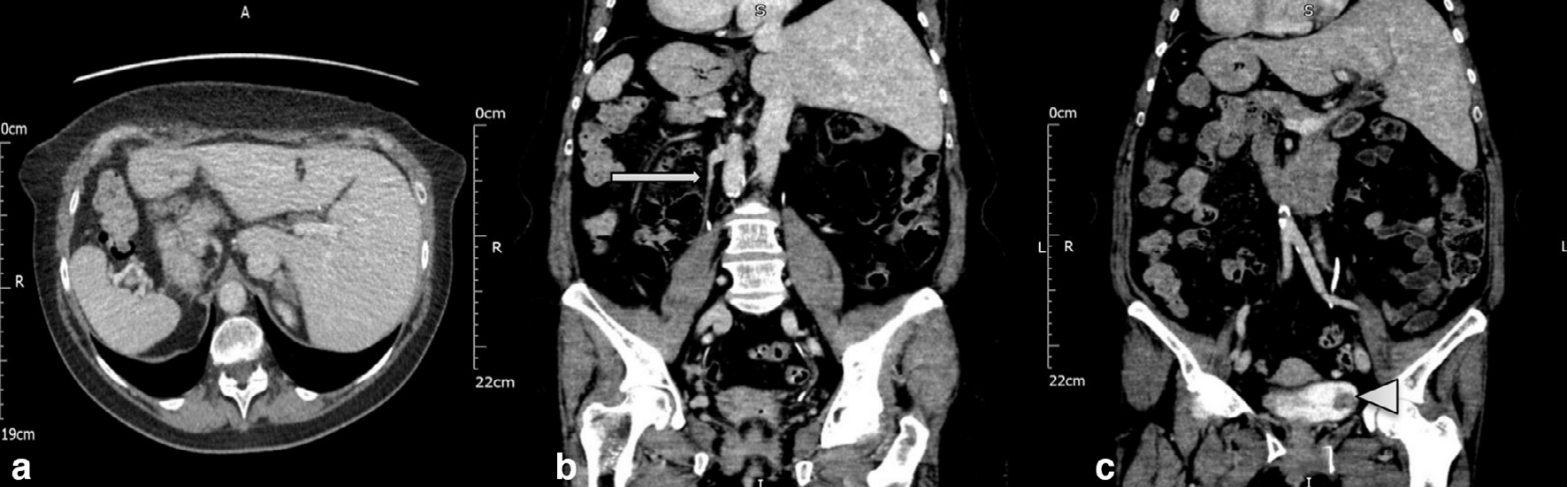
CT in split-bolus excretory phase shows (a) liver, IVC on the left and spleen on the right (b) right gonadal vein opening into the right renal vein (arrow) and (c) tumour in the left lateral wall of the urinary bladder (arrowhead). IVC, inferior vena cava.
